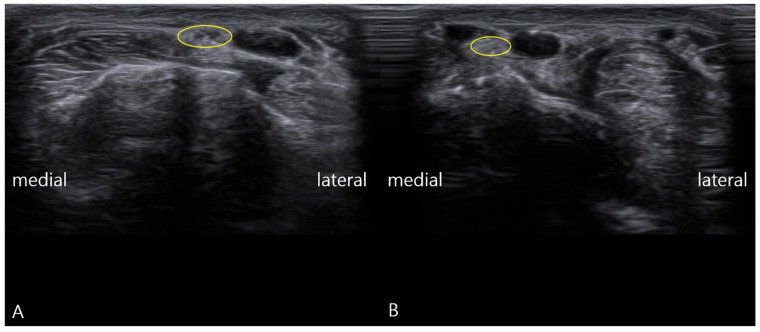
Transverse ultrasound images of the superficial branch of the radial nerve (SBRN): (A) pathologic left wrist showing fascicular thickening of the nerve (yellow circle); (B) healthy right wrist with normal fascicle size (yellow circle).
